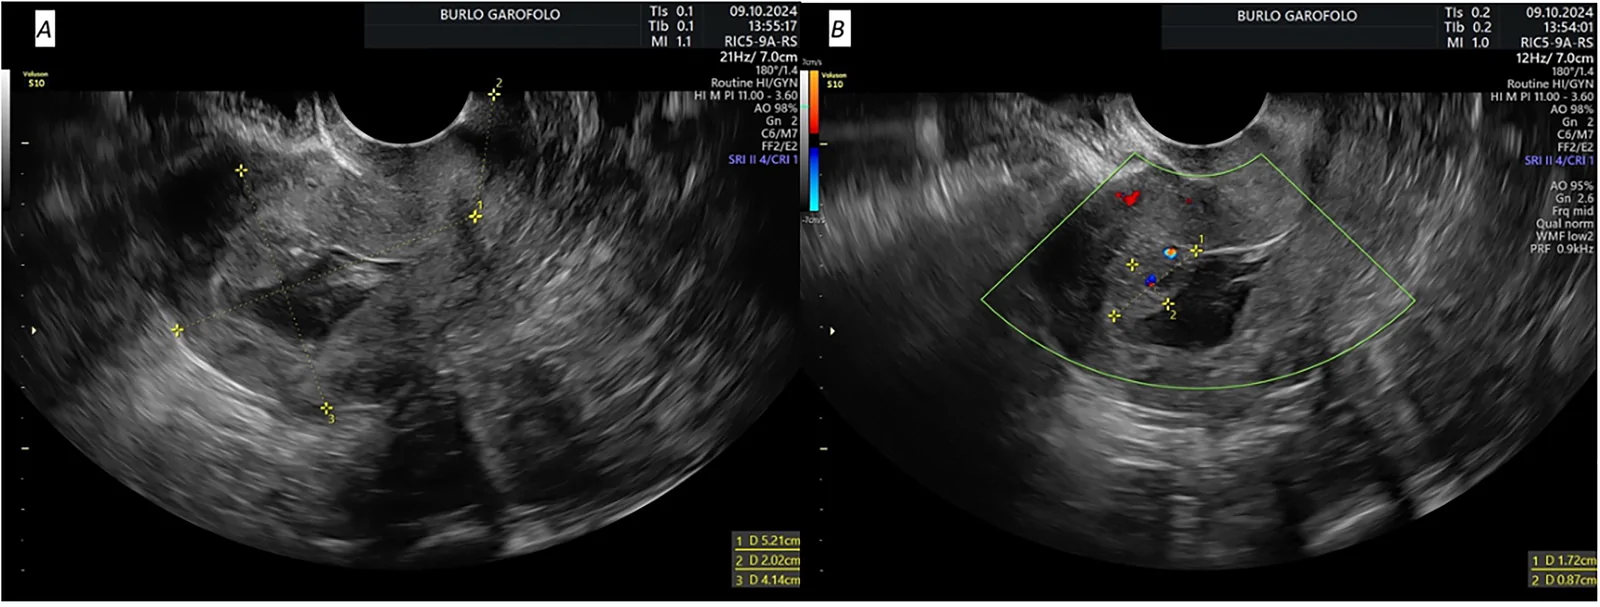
(A) Sonographic appearance of UTROSCT. (B) Measurements of the intracavitary lesion applying Power Doppler.
